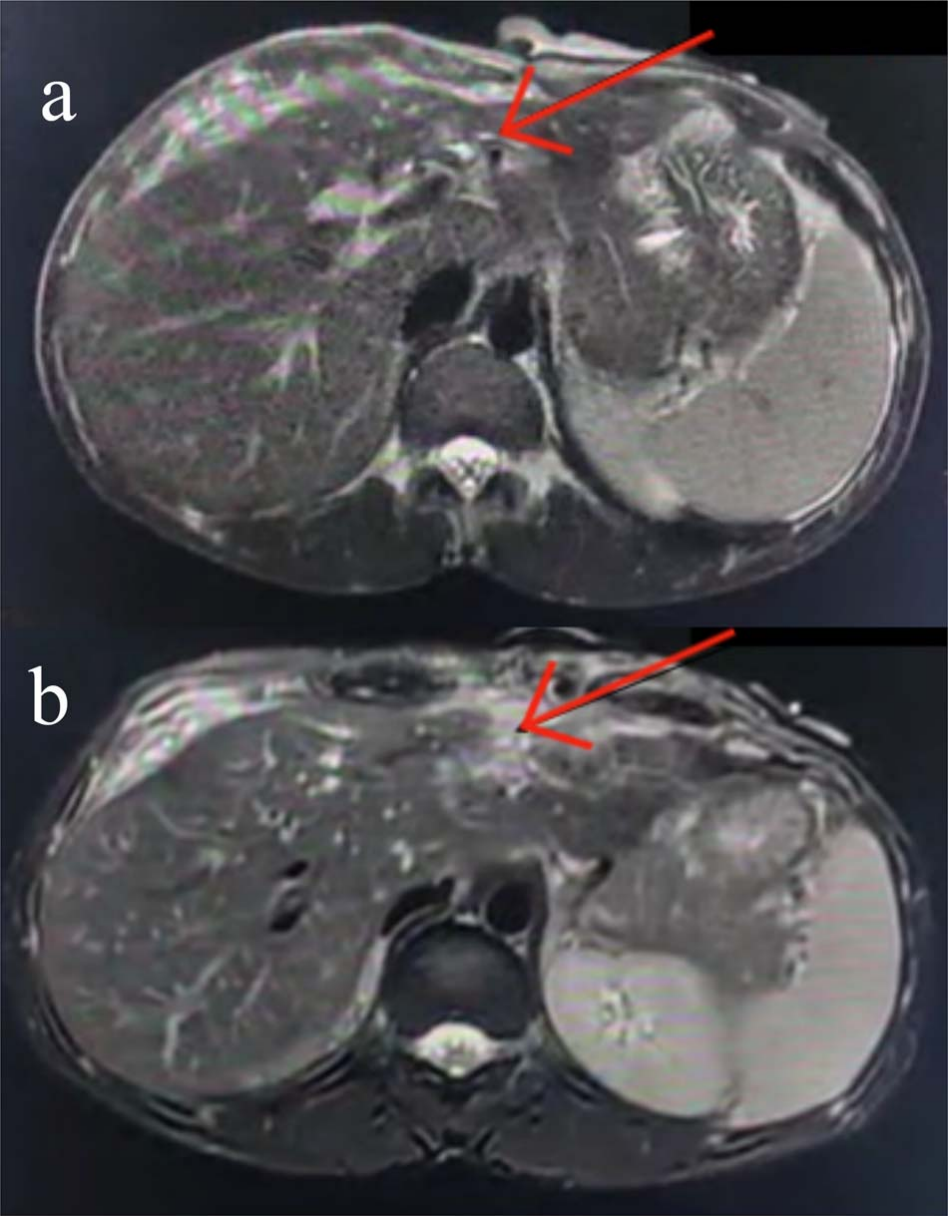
Axial contrast-enhanced abdominal CT images. (a) Subtle injury to the intrahepatic biliary radicals in the left hepatic lobe. (b) Linear laceration on the anterior surface of the liver.
